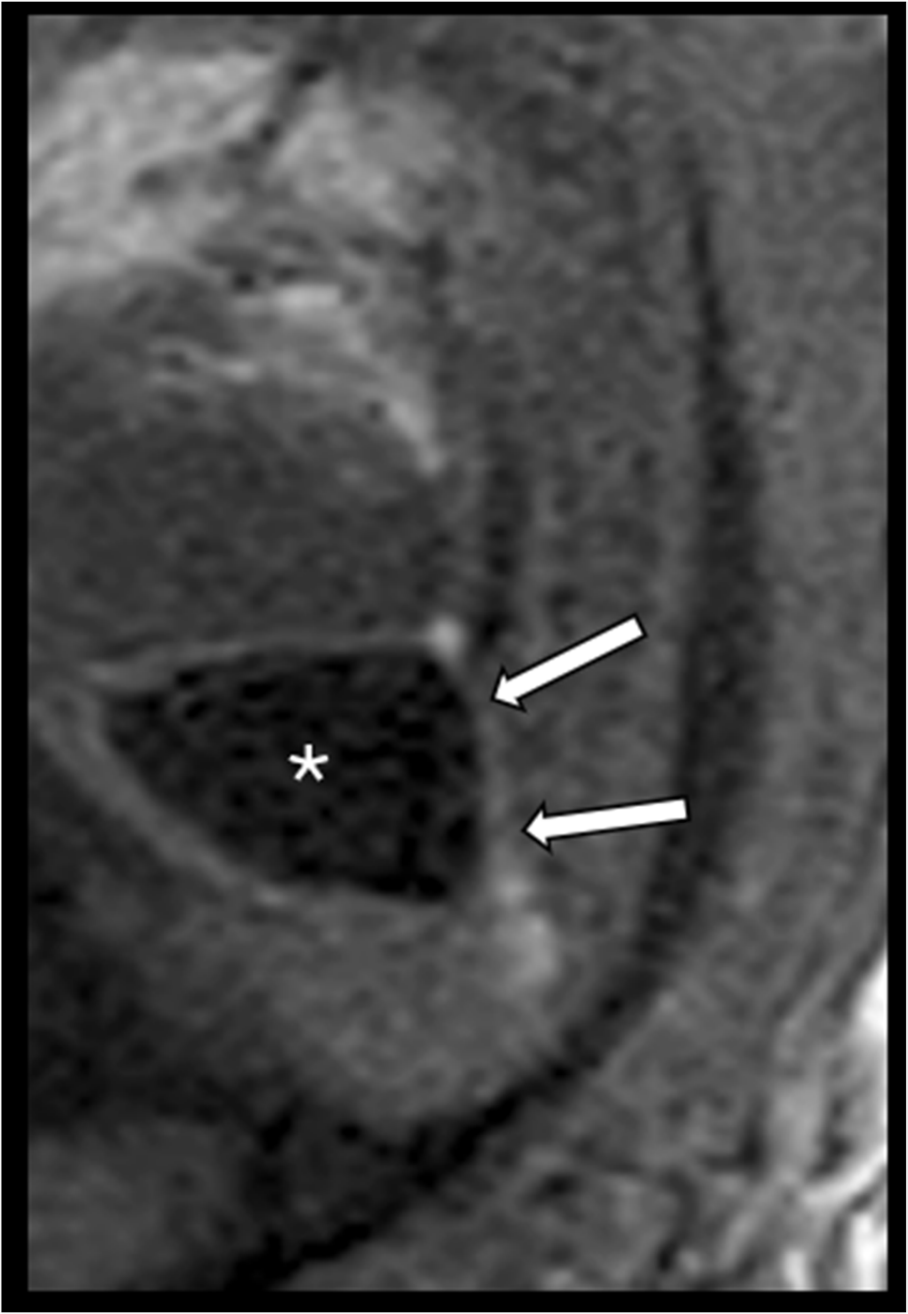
T1-weighted prenatal MRI image sagittal to the fetus at 32 weeks of age; a diagnosis of MMIHS was made postnatally. The image depicts extreme microcolon, which contains T1-hyperintense meconium (*arrows*) and a moderately dilated bladder (*asterisk*)
